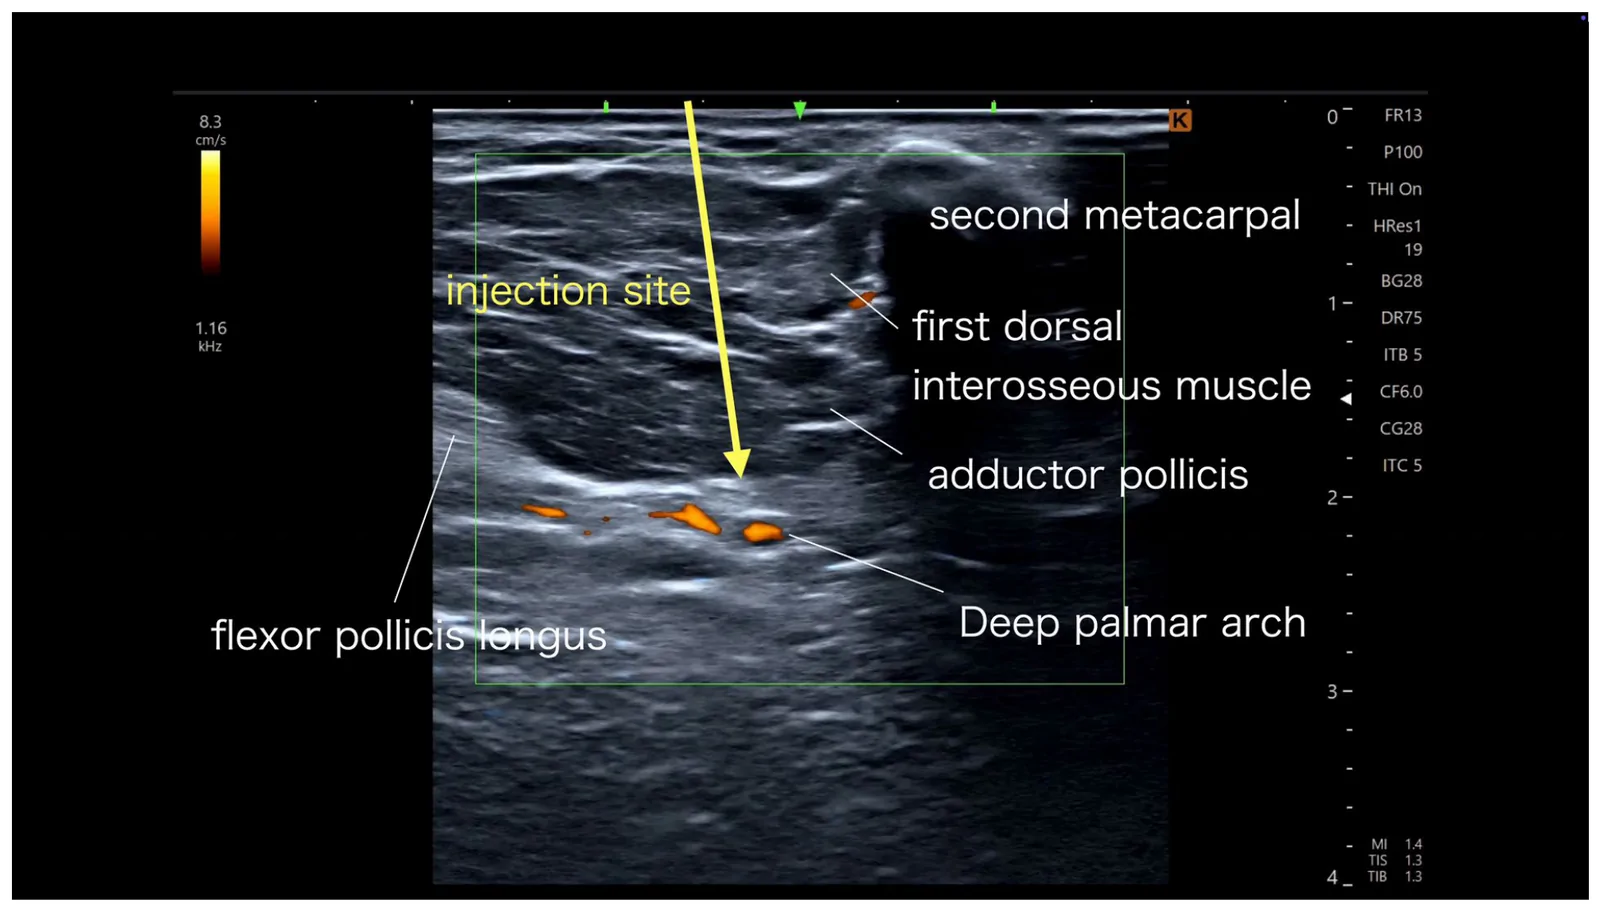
Point 7—first dorsal interosseous/adductor pollicis/deep palmar arch: ultrasound-guided fascia hydrorelease. The green rectangle indicates the color Doppler region of interest, within which the orange signal corresponds to flow in the deep palmar arch. The yellow arrow indicates the needle trajectory toward the injection site.
